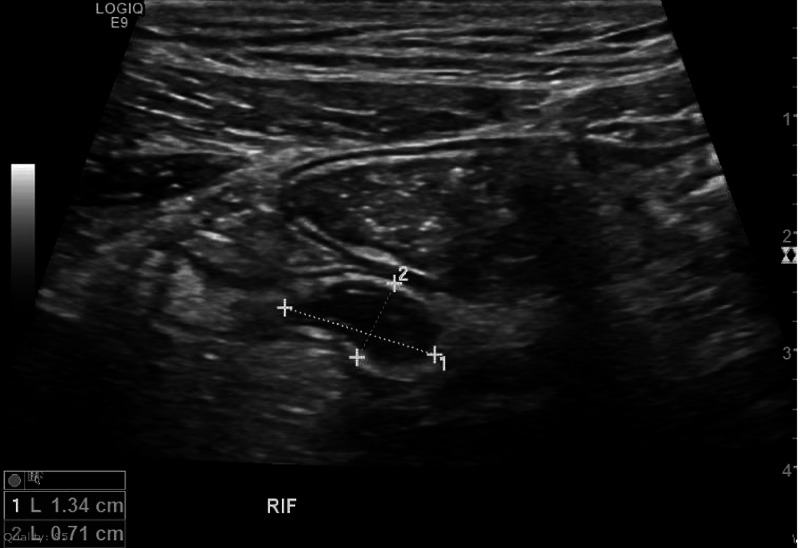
Ultrasound image of the right iliac fossa demonstrating a blind-ending tubular structure distended with fluid measuring 13 mm x 7 mm impressive of obstructed inflamed appendix.
